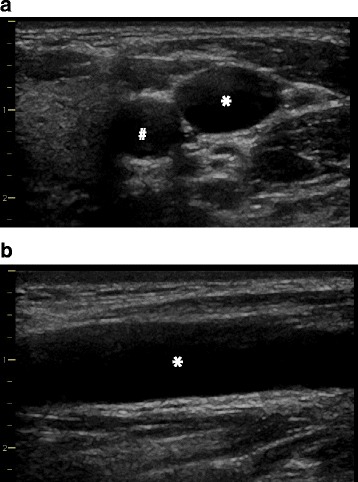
Ultrasound views to identify the anatomy of the target vein. Short-axis (transverse) view (a) and long-axis (longitudinal) view (b) of the right internal jugular vein (*) and its anatomic relation to the carotid artery (#)
